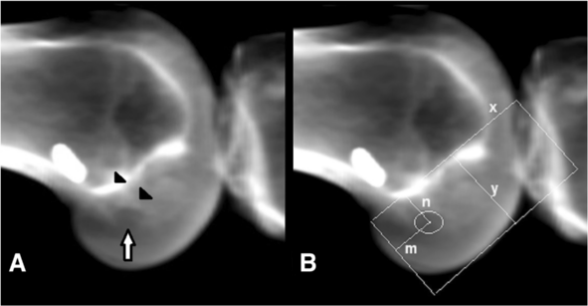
a and b: Digitally reconstructed radiographs showing distal femoral tunnel (arrowheads) and tunnel aperture (arrow) in 1a and Bernard and Hertel grid in 1b
